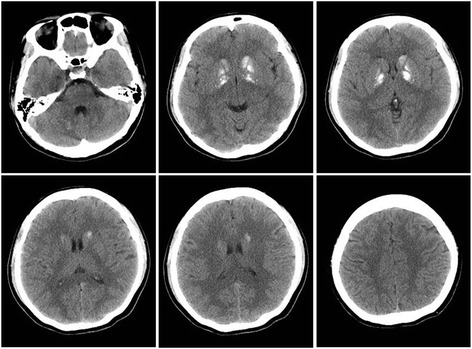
Computed tomography (CT) of a daughter with brain calcification. Symmetric calcifications in bilateral basal ganglia, caudate nucleus, subcortical white matter and cerebellum
